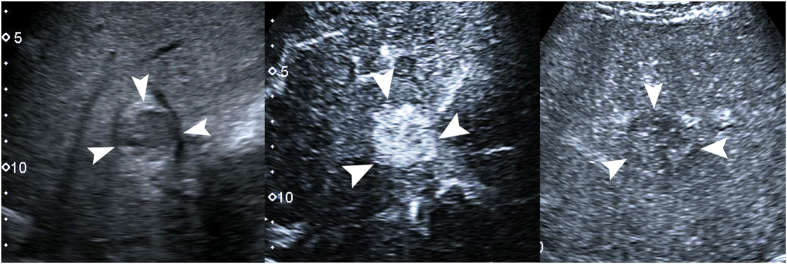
Hepatocellular carcinoma in a 74-year-old man. (a) A 2.9-cm lesion (arrows) in the liver shows hypoechogenicity on conventional ultrasound. (b) Contrast- enhanced ultrasound shows hyperenhancing (arrow) in the lesion 32 s after contrast agent administration. (c) The lesion (arrows) shows hypoenhancement in comparison with adjacent liver tissue 139 s after contrast agent administration.
